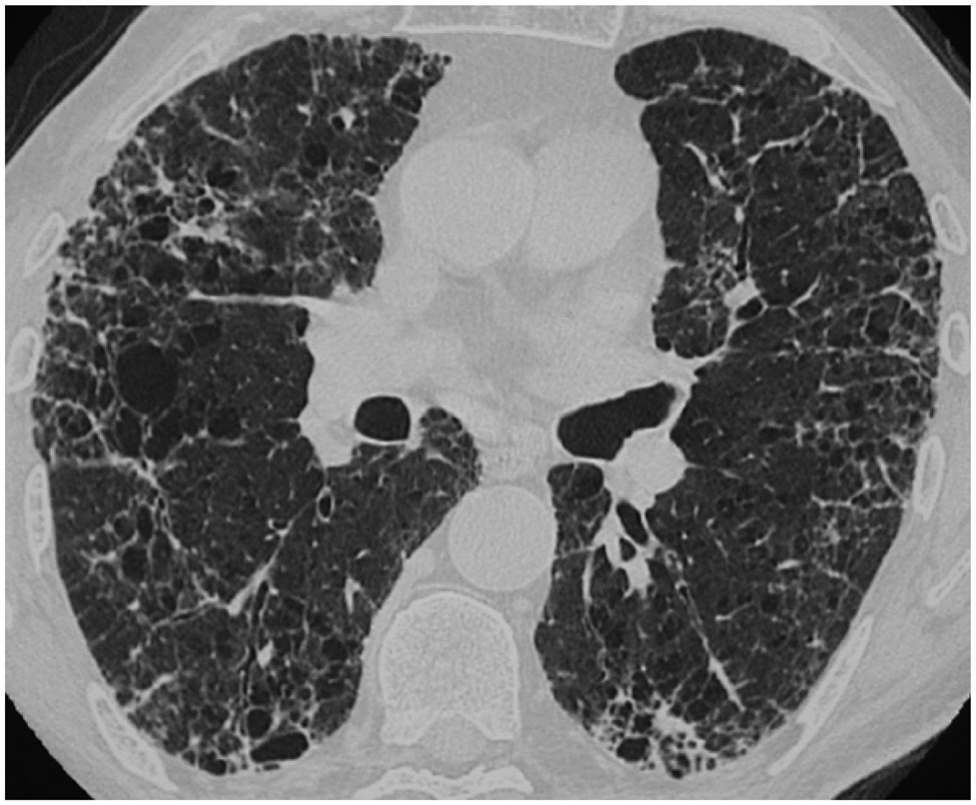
UIP-like comorbidity in a patient with sarcoidosis. CT scan shows a UIP-like pattern, with reticulations, bronchiectasis, and honeycombing with a basal and peripheral predominance in a patient with confirmed sarcoidosis.
